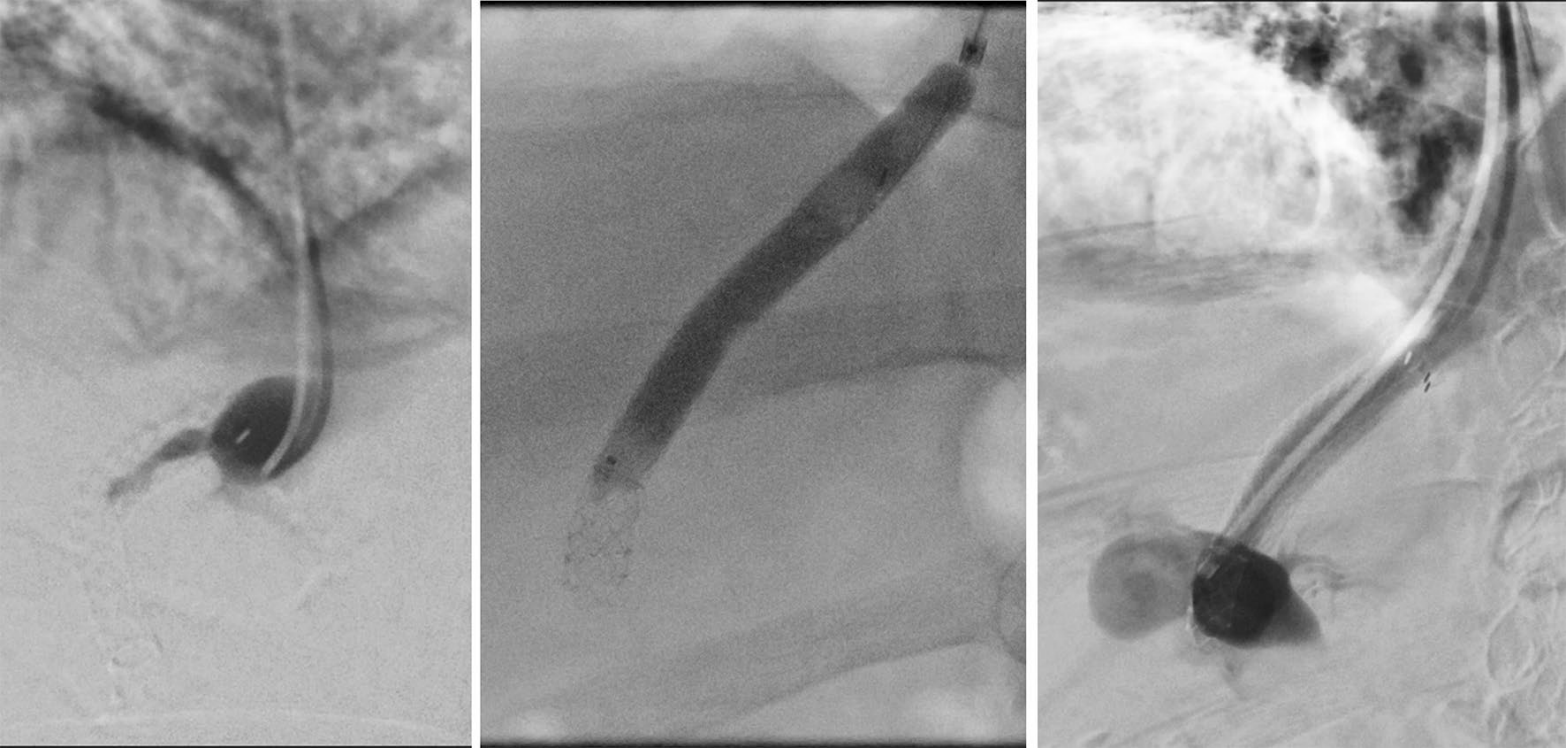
TIPS occlusion. Angiographic evidence of TIPS occlusion at day 23 postinterventional. The patient was previously readmitted with hydropic decompensation and sonographic evidence of early TIPS occlusion. The patency of the TIPS tract was successfully restored by balloon PTA.
